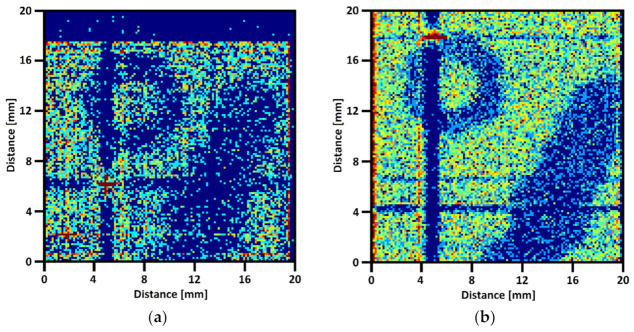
The radiographs of a key and an M6 nut (arrangement shown in Figure 7), measured on the (a) SS-GD; (b) MS readout board.
